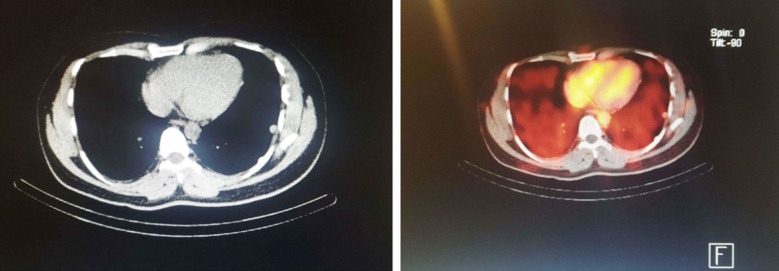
HRCT and 99m-Tc DRBC scan showing pleural based splenic nodules that show uptake of denatured RBCs.
